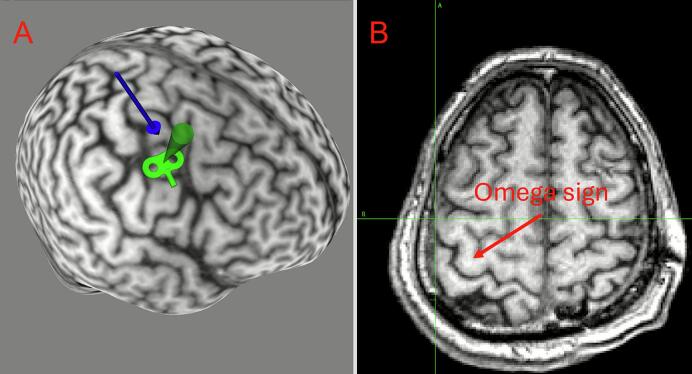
Case 2 neuronavigation target. A. MRI 3D reconstruction with target overlay. The blue arrow marks the M1 hand area; the green shading denotes the stimulation field, targeting the facial motor area. B. An axial image of the stimulation plane. (For interpretation of the references to colour in this figure legend, the reader is referred to the web version of this article.)
